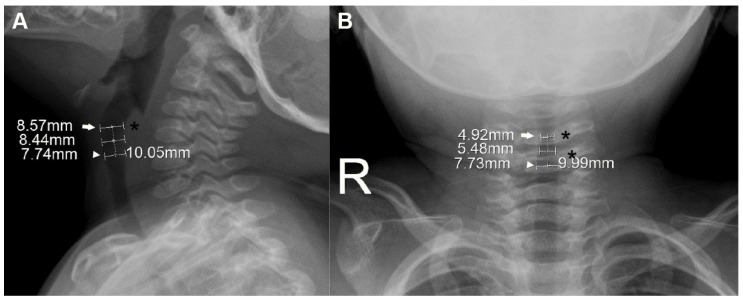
Measurement of laryngeal dimensions in a 15-month-old boy. On the lateral view (A), the glottis (arrow), which was extrapolated from computed tomography-based localization (see Figure S1), is further pointed out by the most protuberant portion of the posterior wall of the hypopharynx (asterisk). On the anteroposterior view (B), the glottis (arrow) is further specified as the narrowest level around the third and fourth cervical vertebrae (asterisks). The cricoid (arrowheads) was defined at a level 10.0 mm caudal to the glottis along the long axis (dotted lines). The subglottis was defined as the midpoint between the two levels. The measured anteroposterior (A) and transverse (B) diameters are noted at each level.
